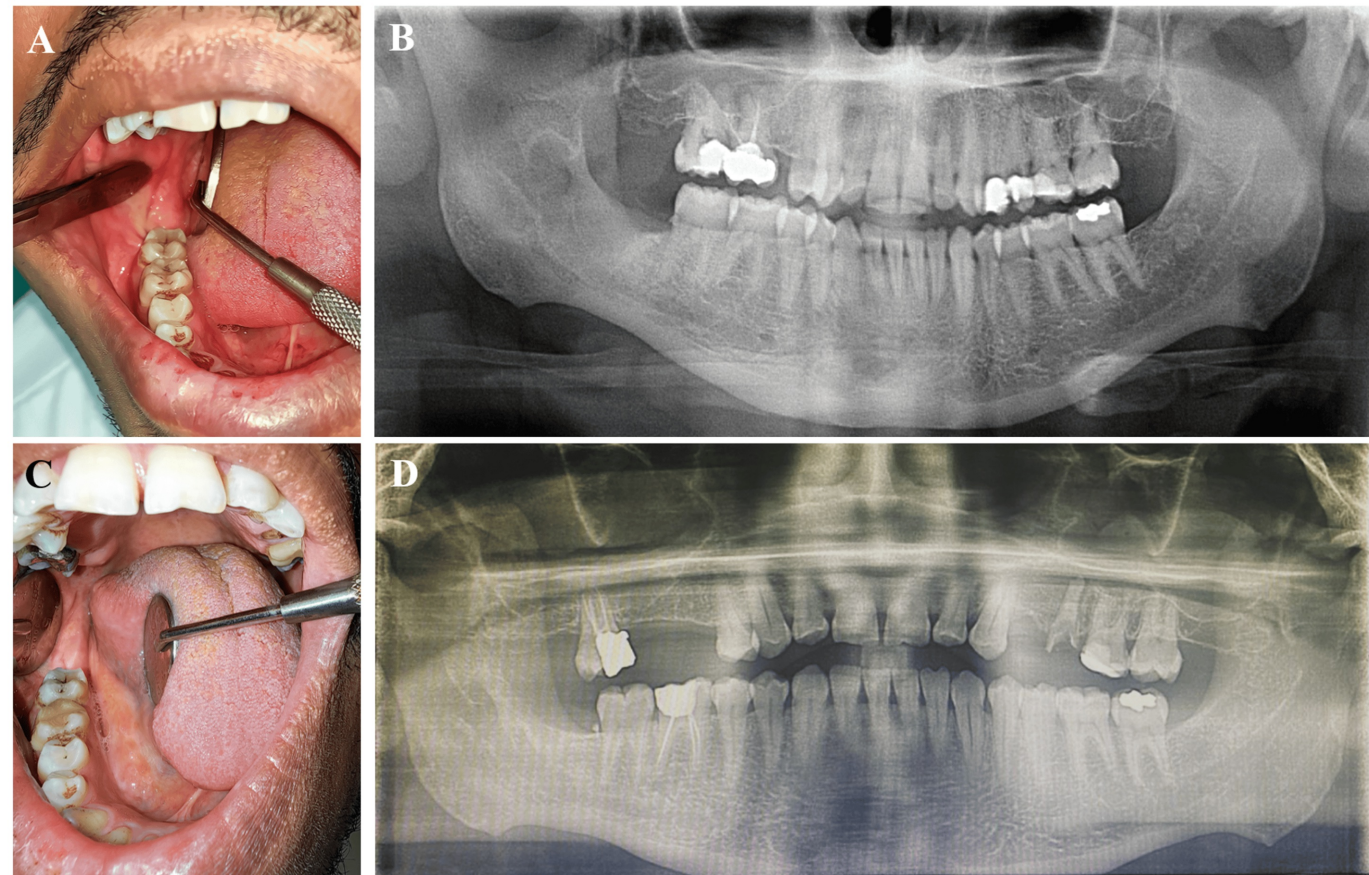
Postoperative intra-oral photographs and panoramic radiographs Four months of follow-up showed complete surgical site healing (A and B), and six years of follow-up showed no signs of recurrence (C and D).
